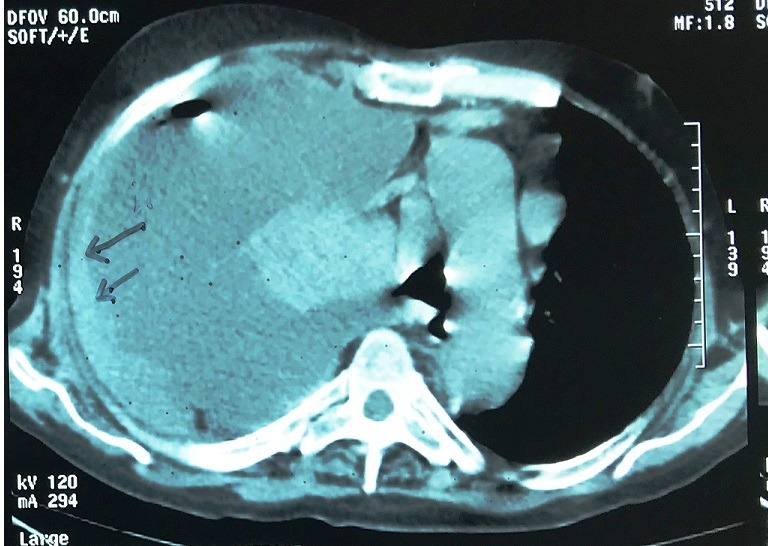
CT-scan showing a prominent thickening of the right pleura
